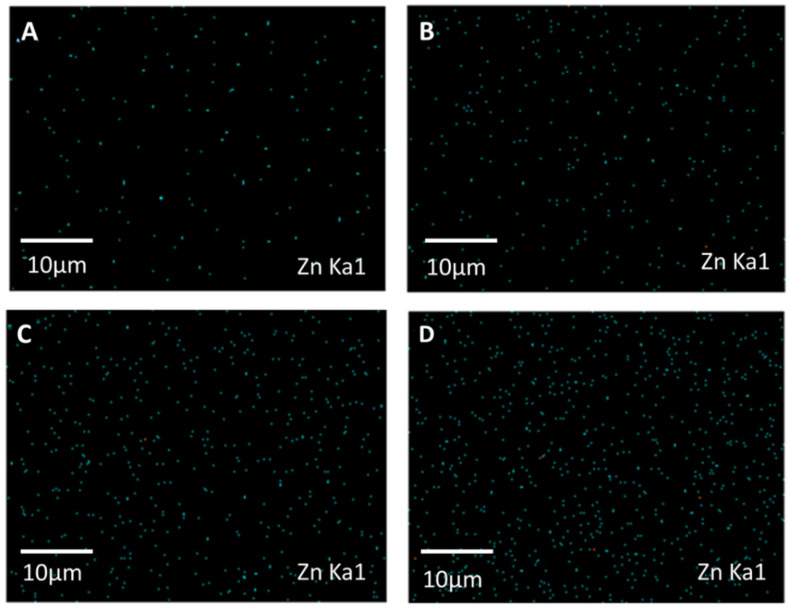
EDX analyses of (A) CTA/CDA-5 wt.% NH_2_-ZIF-8, (B) CTA/CDA-10 wt.% NH_2_-ZIF-8, (C) CTA/CDA-15 wt.% NH_2_-ZIF-8, and (D) CTA/CDA-20 wt.% NH_2_-ZIF-8.
